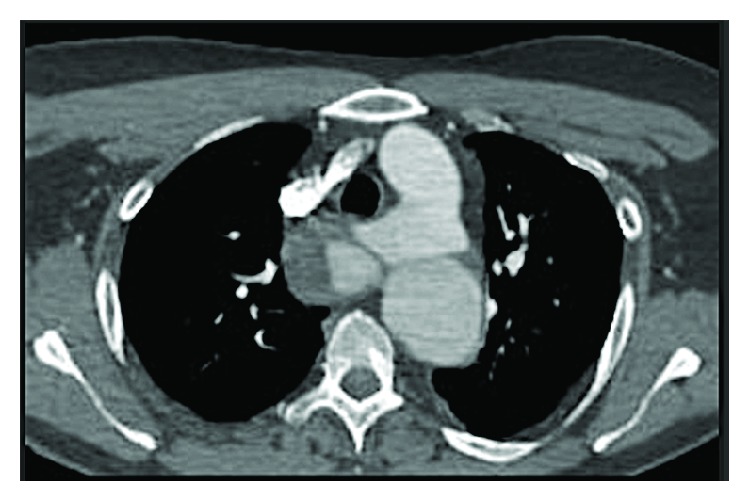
Preoperative chest CT showing type B aortic dissection from the origin of the left subclavian artery.
